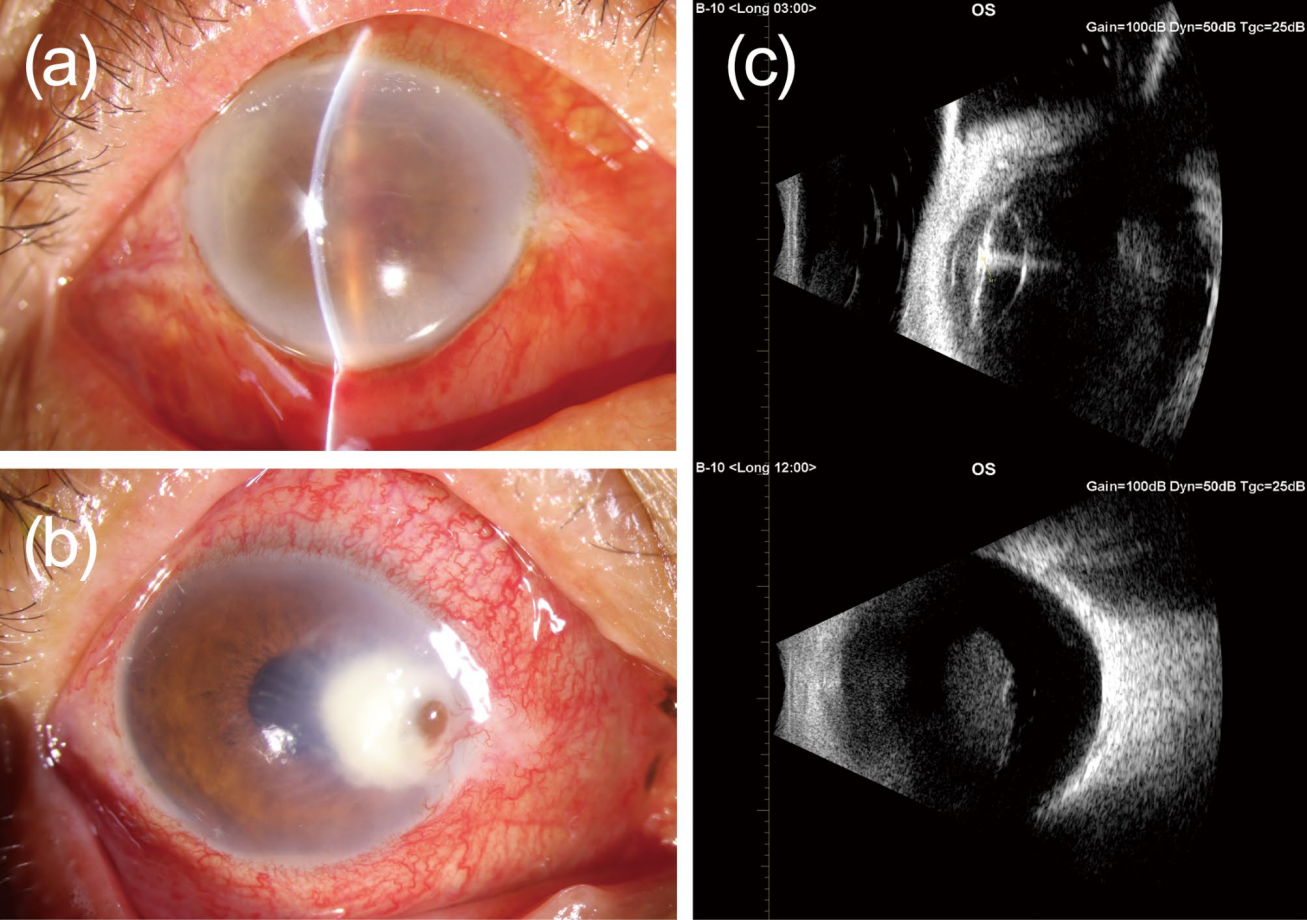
(a) Anterior segment photography of case 6: Conjunctival injection, corneal edema, aqueous flare, hypopyon about 1 mm, and exudation obscuring the pupil is observed in the right eye; (b) Anterior segment photography of case 12: Conjunctival injection, ulcerative lesion about 5 × 5 mm at nasal limbus, corneal perforation with iris prolapse, neovascularization, aqueous flare, hypopyon, and posterior synechia is observed in the right eye; (c) B-scan ultrasonography of case 10: Vitreous opacities in the left eye
